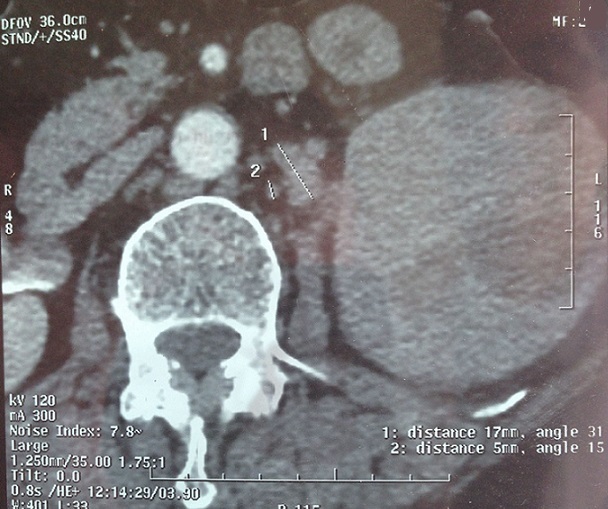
Abdominal Computed tomography scan showed Several small lymphadenopathy in the right hilar area
